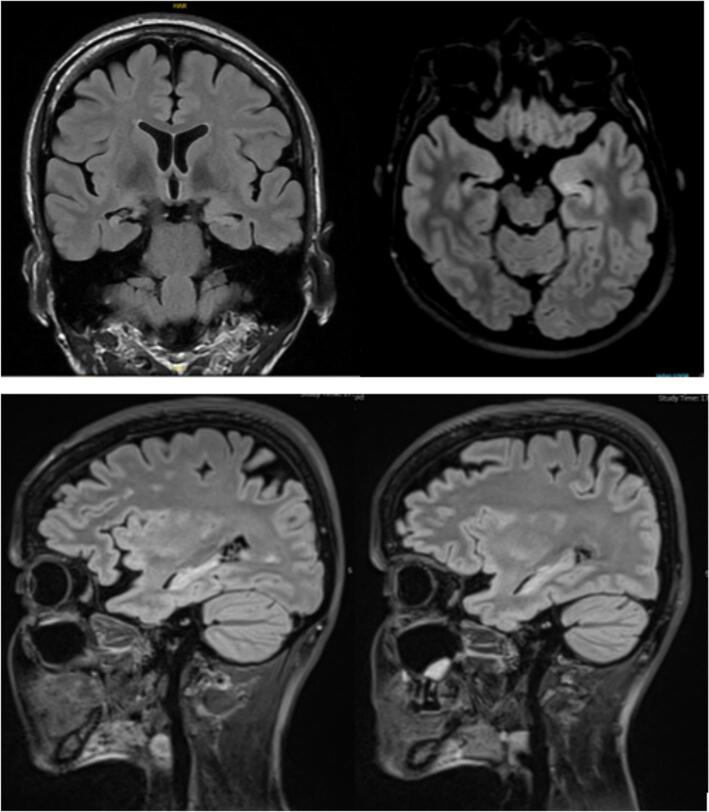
Coronal, axial and sagittal cuts of MRI T2 FLAIR showing bilateral mesial temporal sclerosis more pronounced on the right side.
